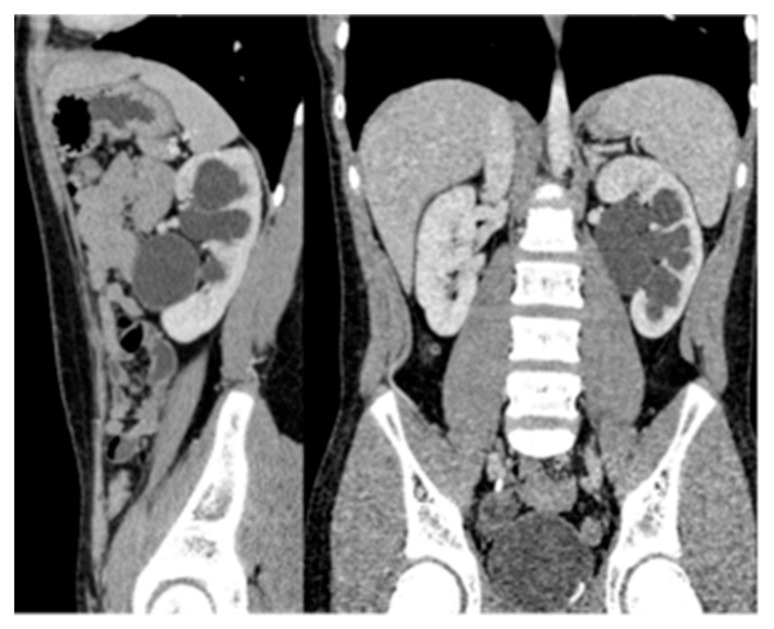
CT scan showing left ureteropelvic junction obstruction.
